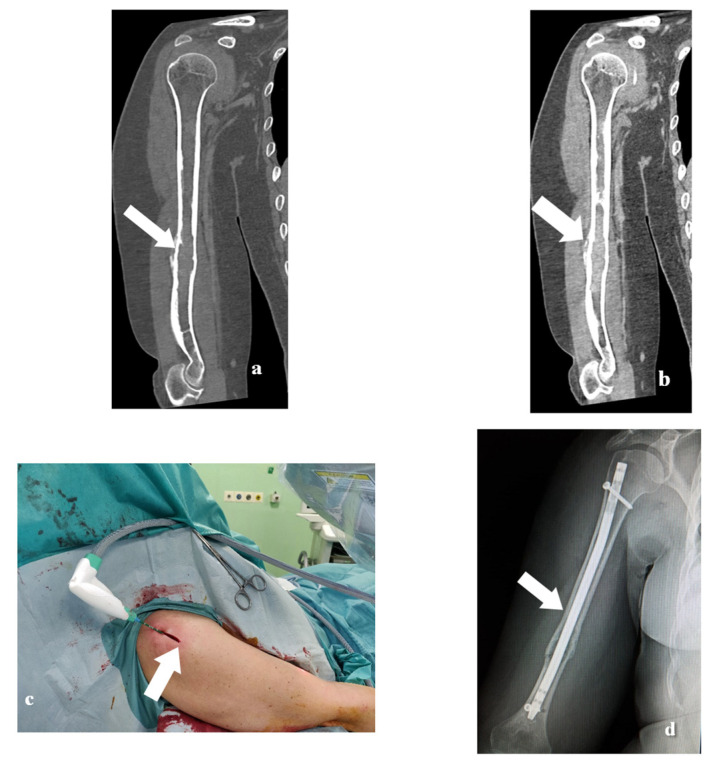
A 56-year-old female patient with a right humerus metastasis from breast cancer (a–d). Coronal CT with bone window (a) and soft tissue window (b) show the presence of a lytic lesion in the middle portion of the humerus. The panel (c) shows the treatment whereas the panel (d) shows the presence of the endoprosthetic replacement.
